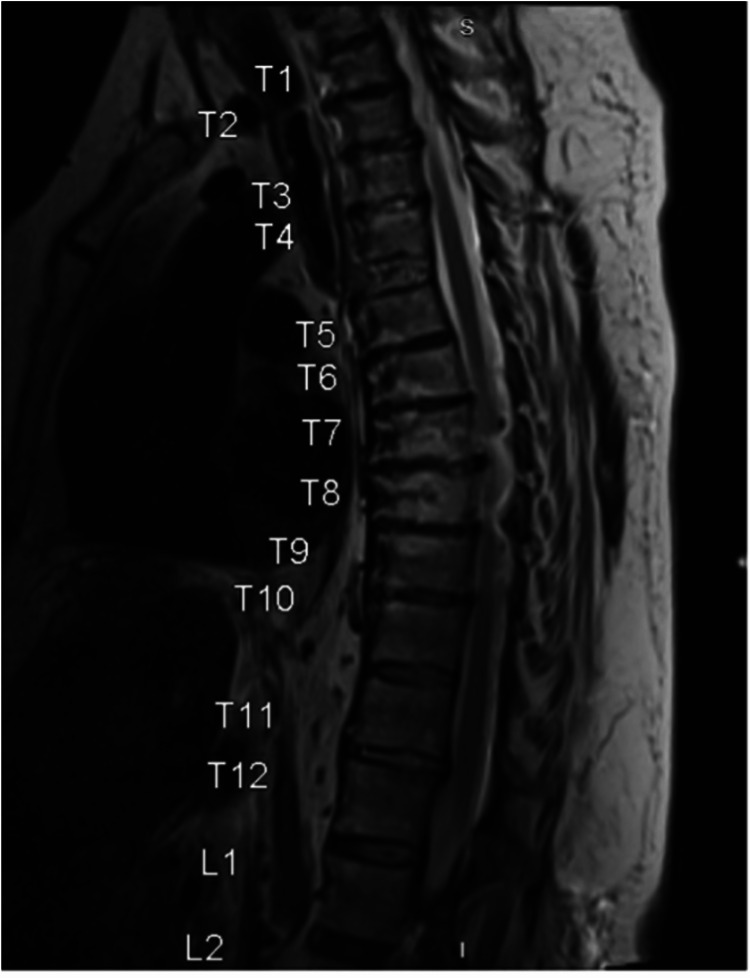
T2-weighted sagittal MRI T4/5 disc disruption as well as anterior longitudinal ligamentous and ligamentum flavum disruption without spinal cord injury
